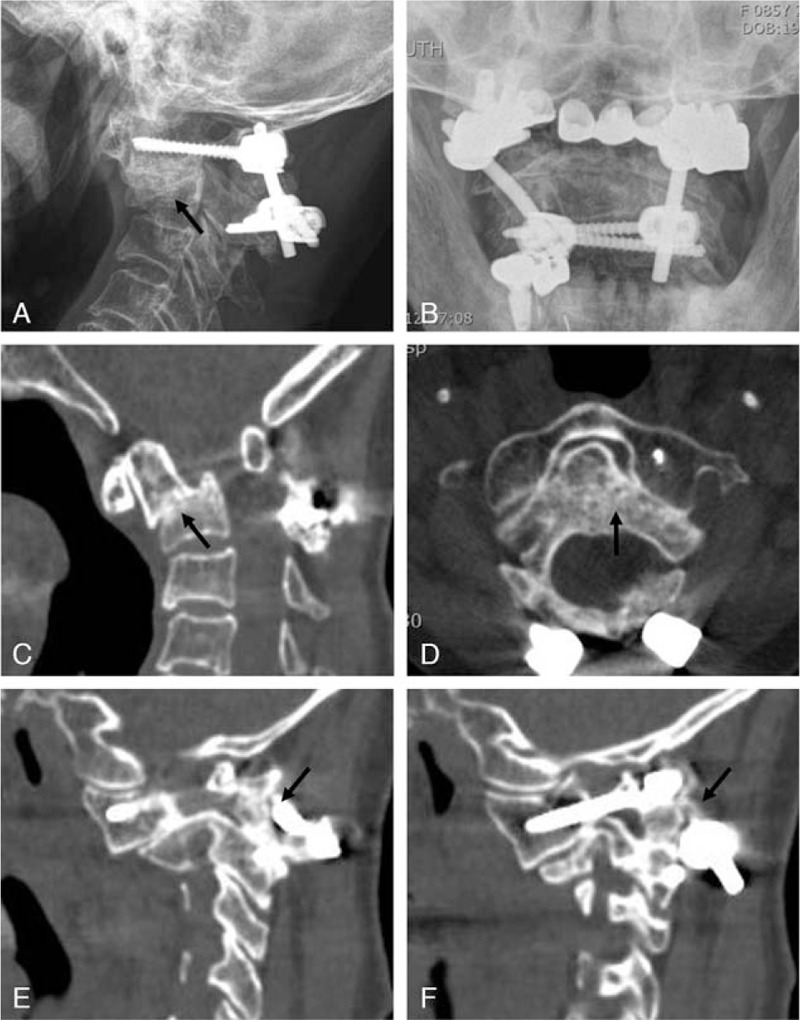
One year after posterior C1-2 fusion surgery, lateral radiograph (a) and midline sagittal computed tomography (CT) (c) show the union (dark arrows) of the type IIA dens fracture in a kyphotic state. An open-mouth view (b) and axial CT scan (d) reveals the union of the type IIA dens fracture fragment at an anterolateral rotatory angulated state (dark arrow). The right (e) and left (f) parasagittal CT scans demonstrate the fracture union of both pars interarticularis (type I Hangman fracture) and left transverse foramen (dark arrows).
